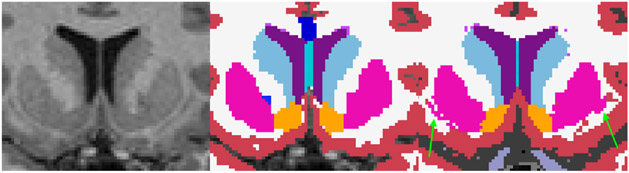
An example segmentation of the putamen structure. Left panel shows a region of MRI T1w image where putamen and claustrum are visible; center panel shows the result of ASEG segmentation; right panel shows the result of SAMSEG segmentation with green arrows pointing to the parts of claustrum structure which are segmented as putamen.
